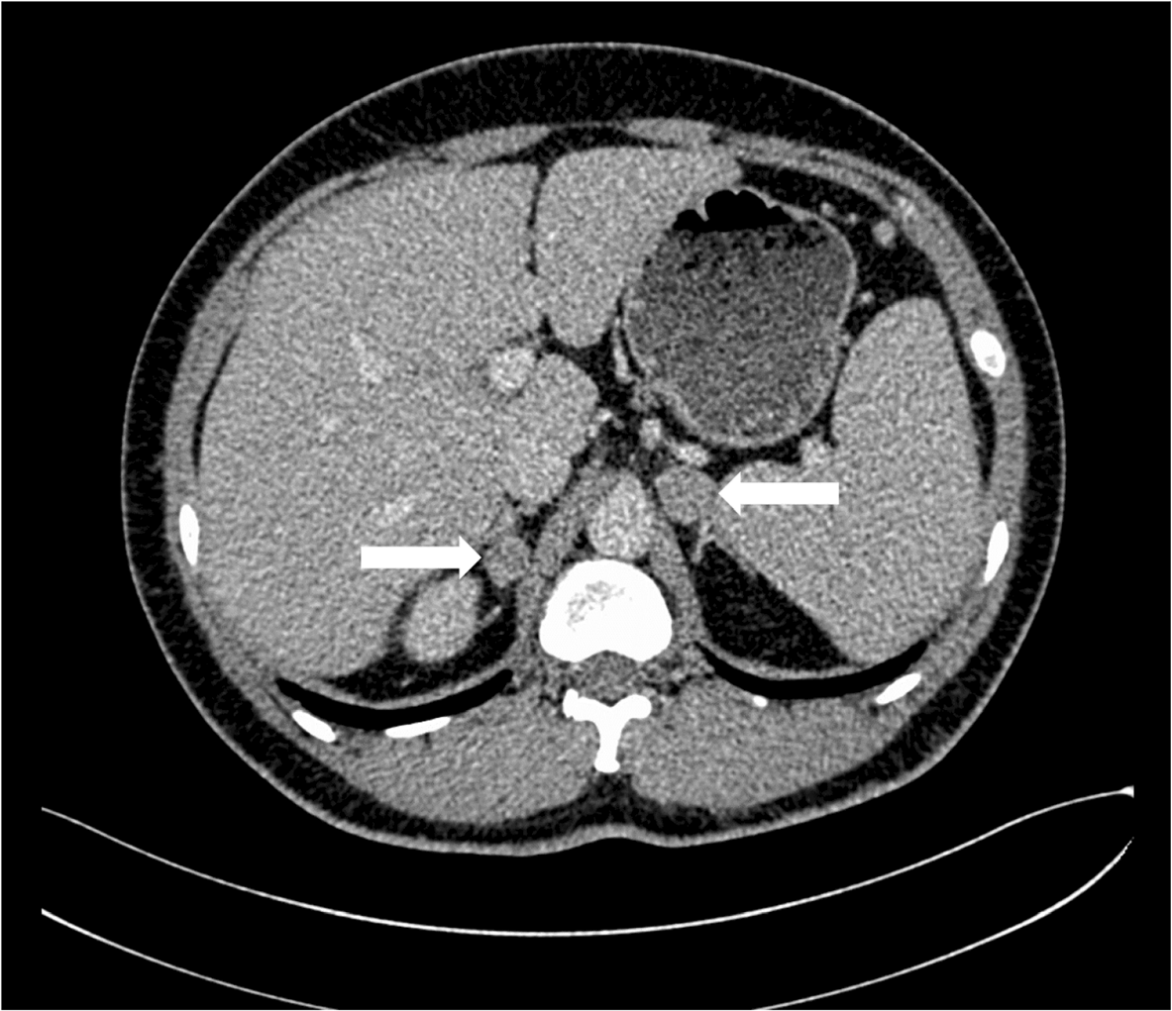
Adrenal computed tomography (CT) image showing a 19 × 14 mm right adrenal tumor and a 25 × 15 mm left adrenal tumor (arrows)
